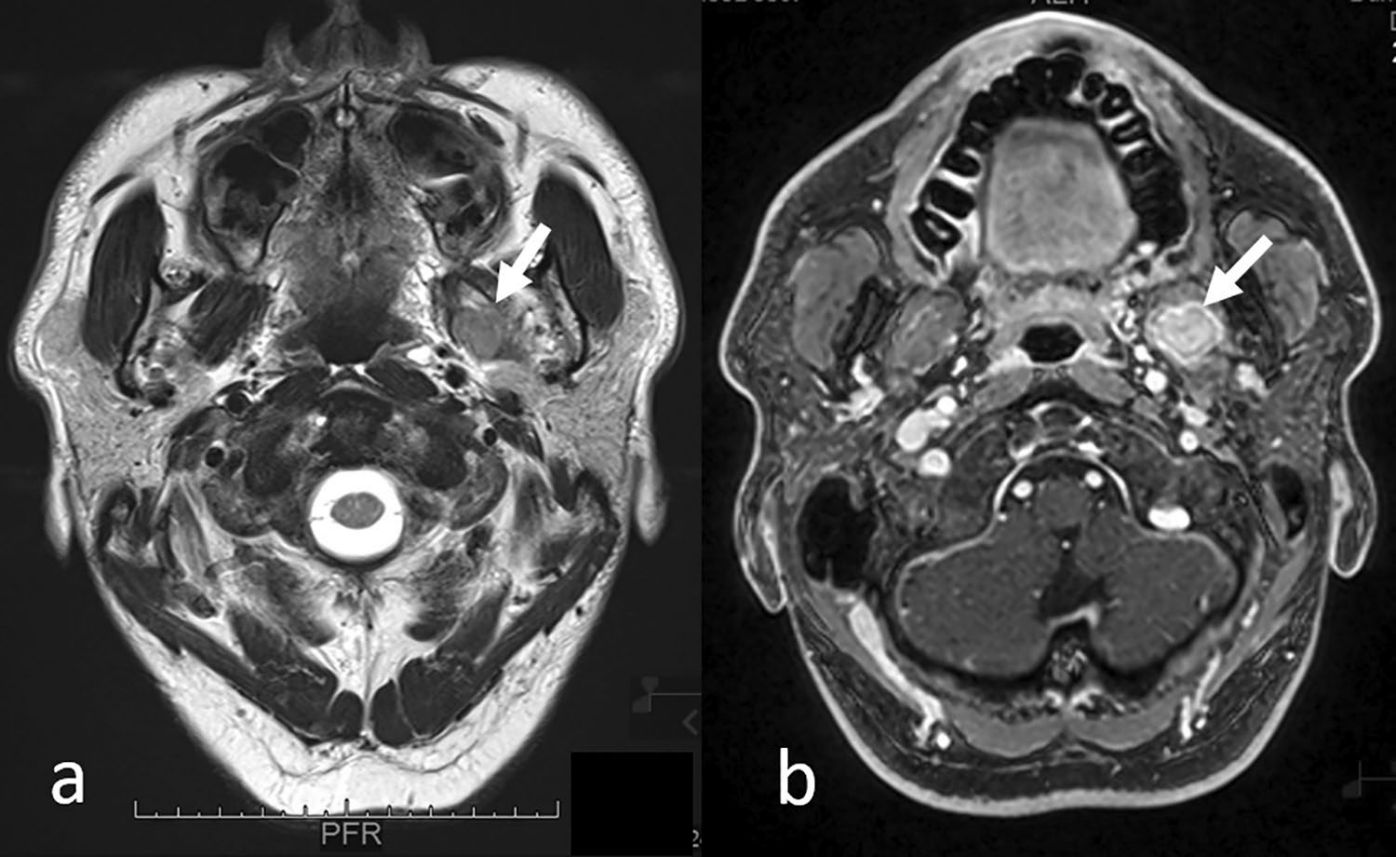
Well-defined left pterygoid muscle metastasis in a patient with a lung adenocarcinoma. T2-weighted image a shows the tumor as slightly hyperintense compared to the contralateral pterygoid muscle (arrow). Contrast-enhanced 3D fat-suppressed T1-weighted image b shows homogeneous, well-defined contrast enhancement of the tumor (arrow)
